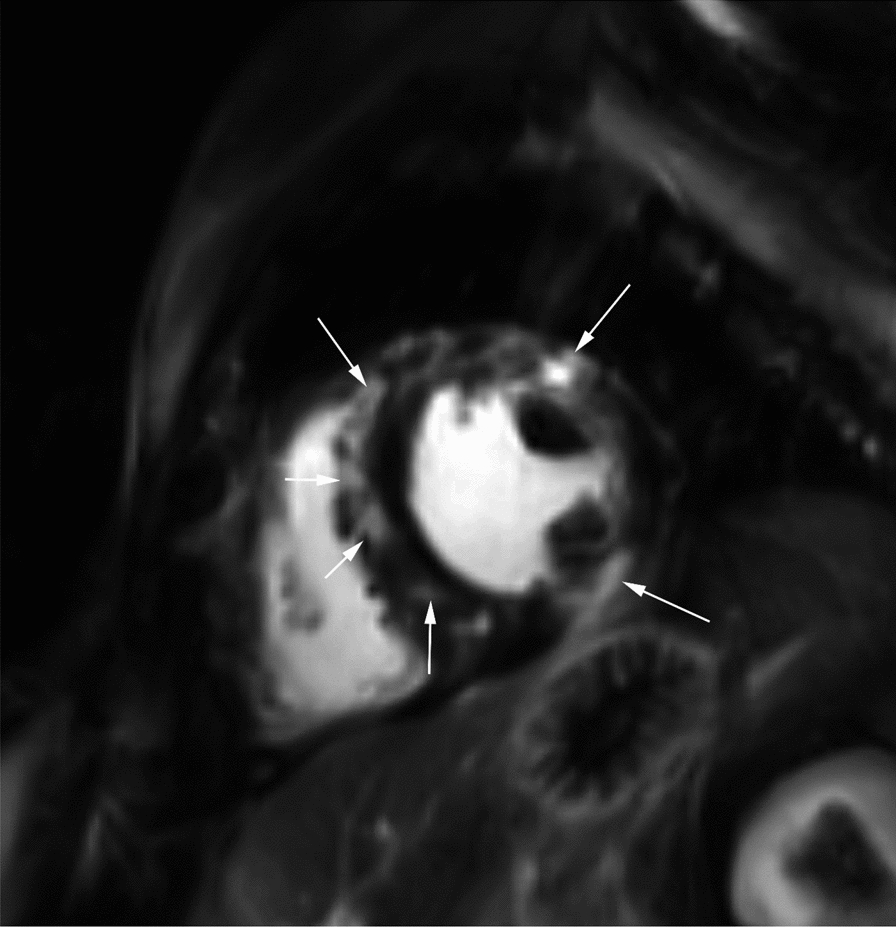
45 year-old male MELAS patient with severe cardiac involvement. MRI PSIR (*Phase-sensitive inversion recovery) *image in short axis orientation 10 min after CE-adminstration. The white arrows mark some of the late-enhancement areas in the ventricular septum and in the inferior and anterior wall of the left ventricle
